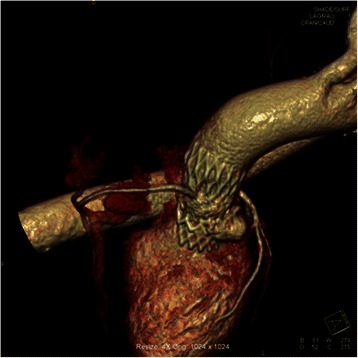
CT scan after the 2nd TAVI. A pseudoaneurysm is located in the area of the left sinus Valsalvae.
